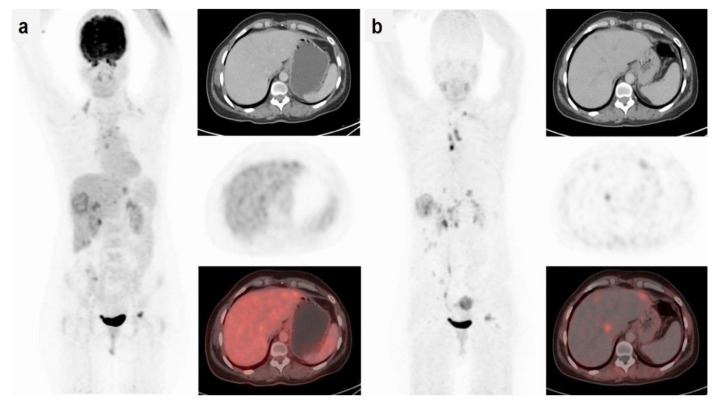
A 52-year-old patient with breast cancer. (a) [^18^F]FDG-PET/CT showed low or no uptake in the hepatic lesions (SUV_max_ = 3.9); (b) [^68^Ga]Ga-FAPI-04-PET/CT showed high uptake (liver metastases SUV_max_ = 9.1) in all lesions. (Reprinted with permission from Ref. [29], 2021, Springer Nature.)
